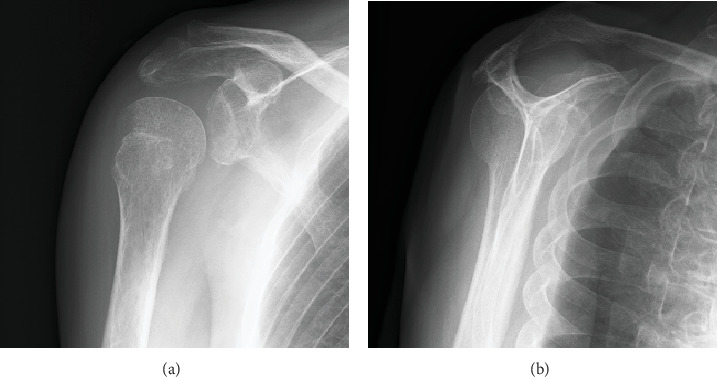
Initial plain radiographic images on first referral to our hospital. (a) An anteroposterior radiograph showing a light bulb-like appearance of the humeral head with a malunited greater tuberosity. (b) A scapular Y view showing the posterior shift of the humeral head.
